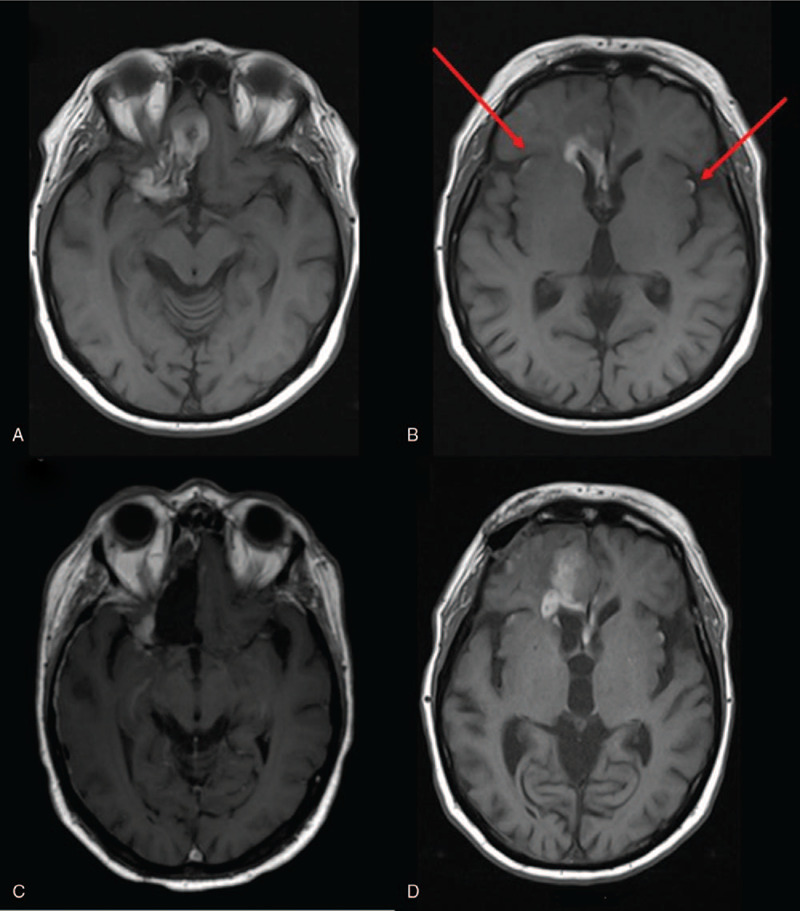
Brain MRI: on axial images hyperintense nodular tumor in right frontal lobe preoperatively in November 2016 (A) and leptomeninegeal dissemination marked with red arrows (B), postoperative residual tumor (C) and tumor progression in March 2019 (D).
